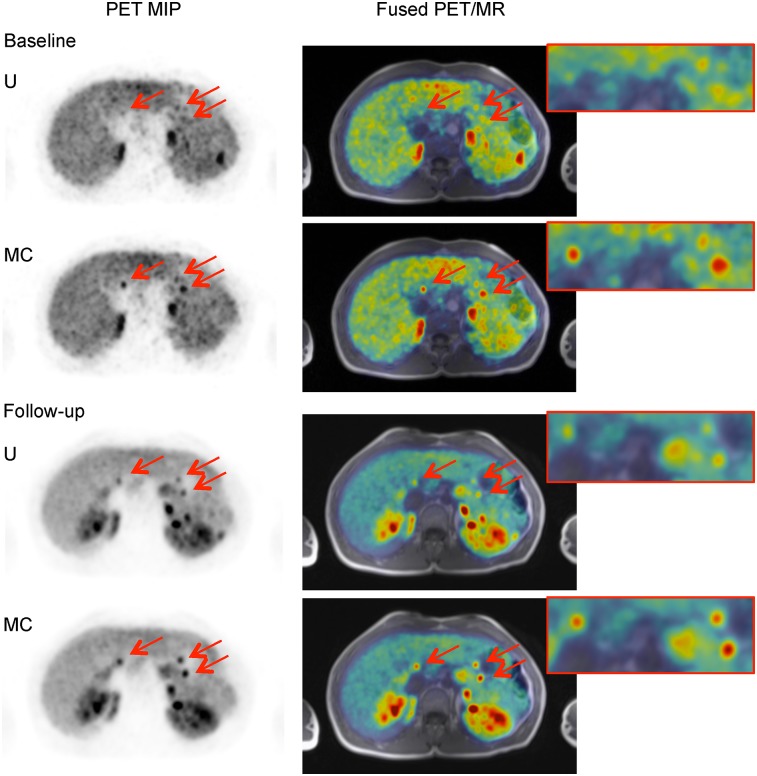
Case 2. Axial uncorrected (U) and motion-corrected (MC) PET maximum-intensity projection (MIP) images showing 3 pancreas lesions (arrows) at baseline and follow-up, along with T1-weighted Dixon volumetric interpolated breath-hold examination PET/MR images.
